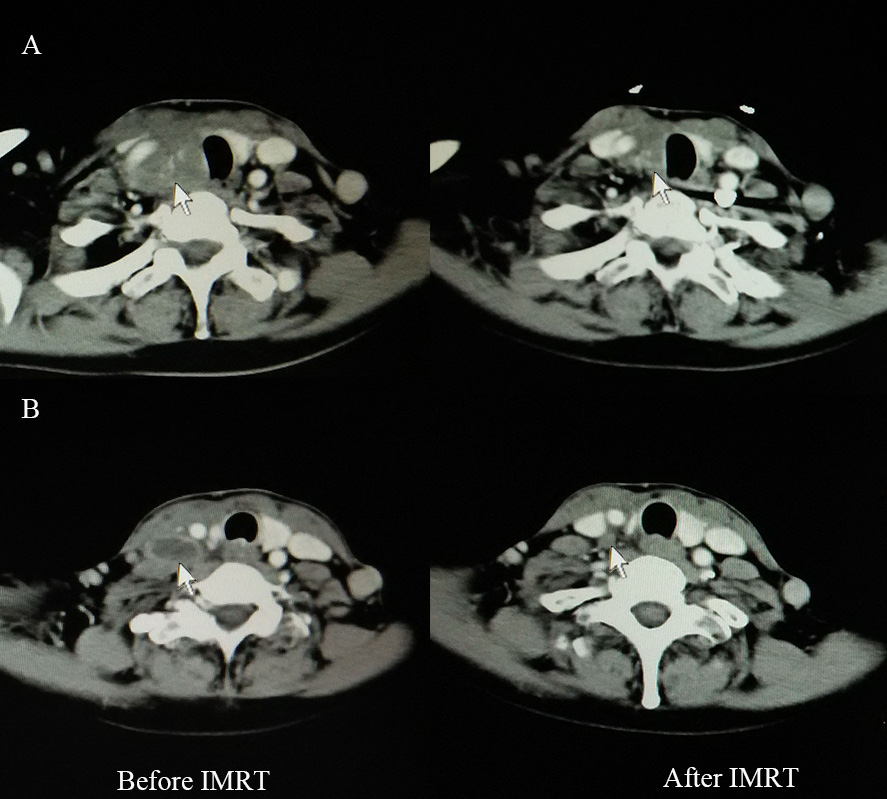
Computed tomography findings before (left) and after (right) IMRT for one patient with CASTLE who received palliative resection A. Disease extent for primary tumor (white arrow). B. Disease extent for involved lymph node (white arrow). IMRT, intensity-modulated radiotherapy; CASTLE, carcinoma showing thymus-like differentiation.
